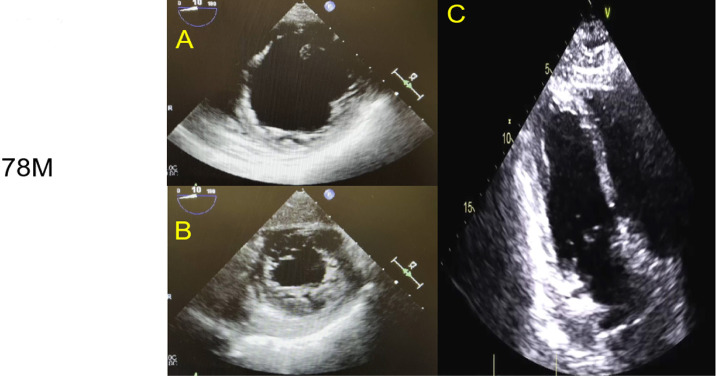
Intraoperative transesophageal echocardiography imaging of a
